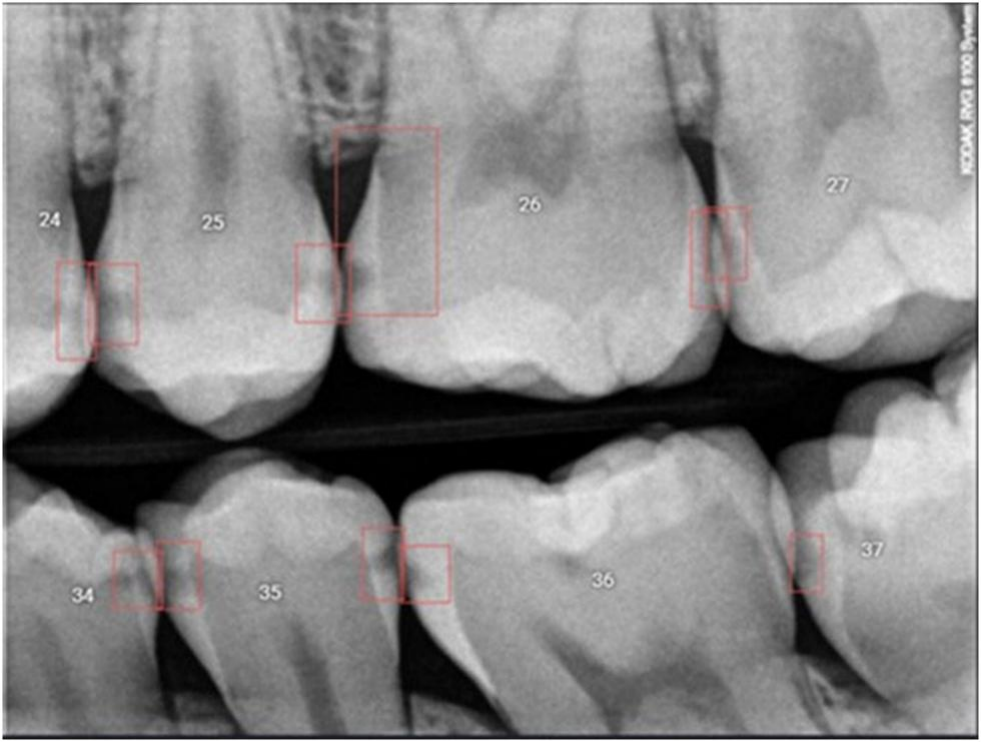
Bitewing radiograph with ML annotations identifying carious sites.
